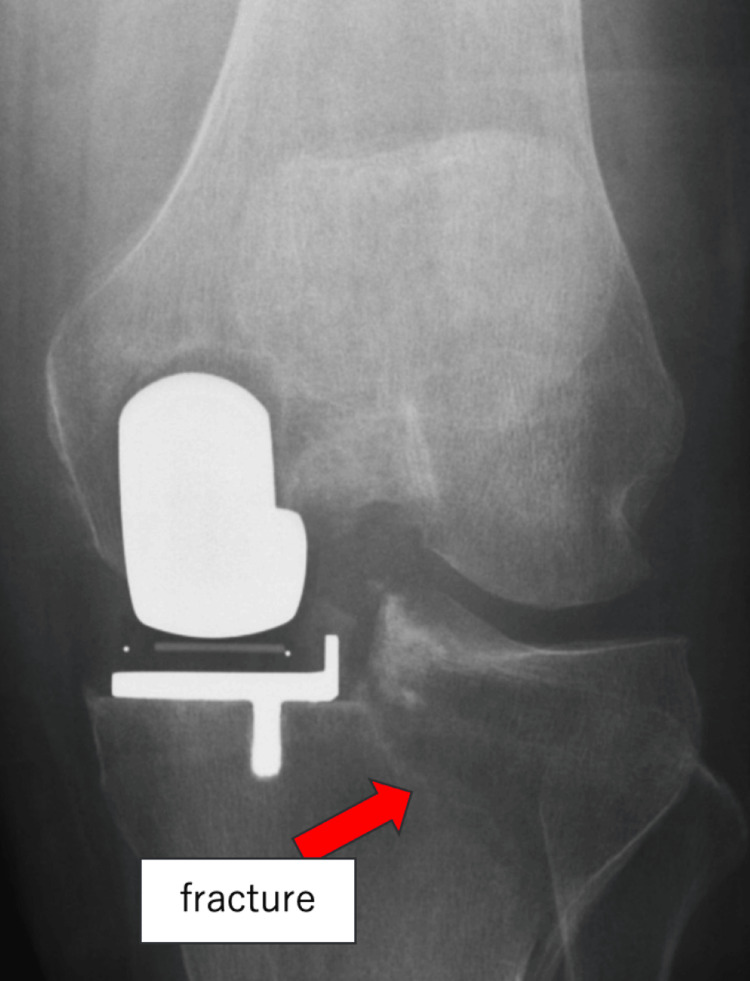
X-ray taken the day after surgery shows a lateral tibial plateau fracture (arrow).
